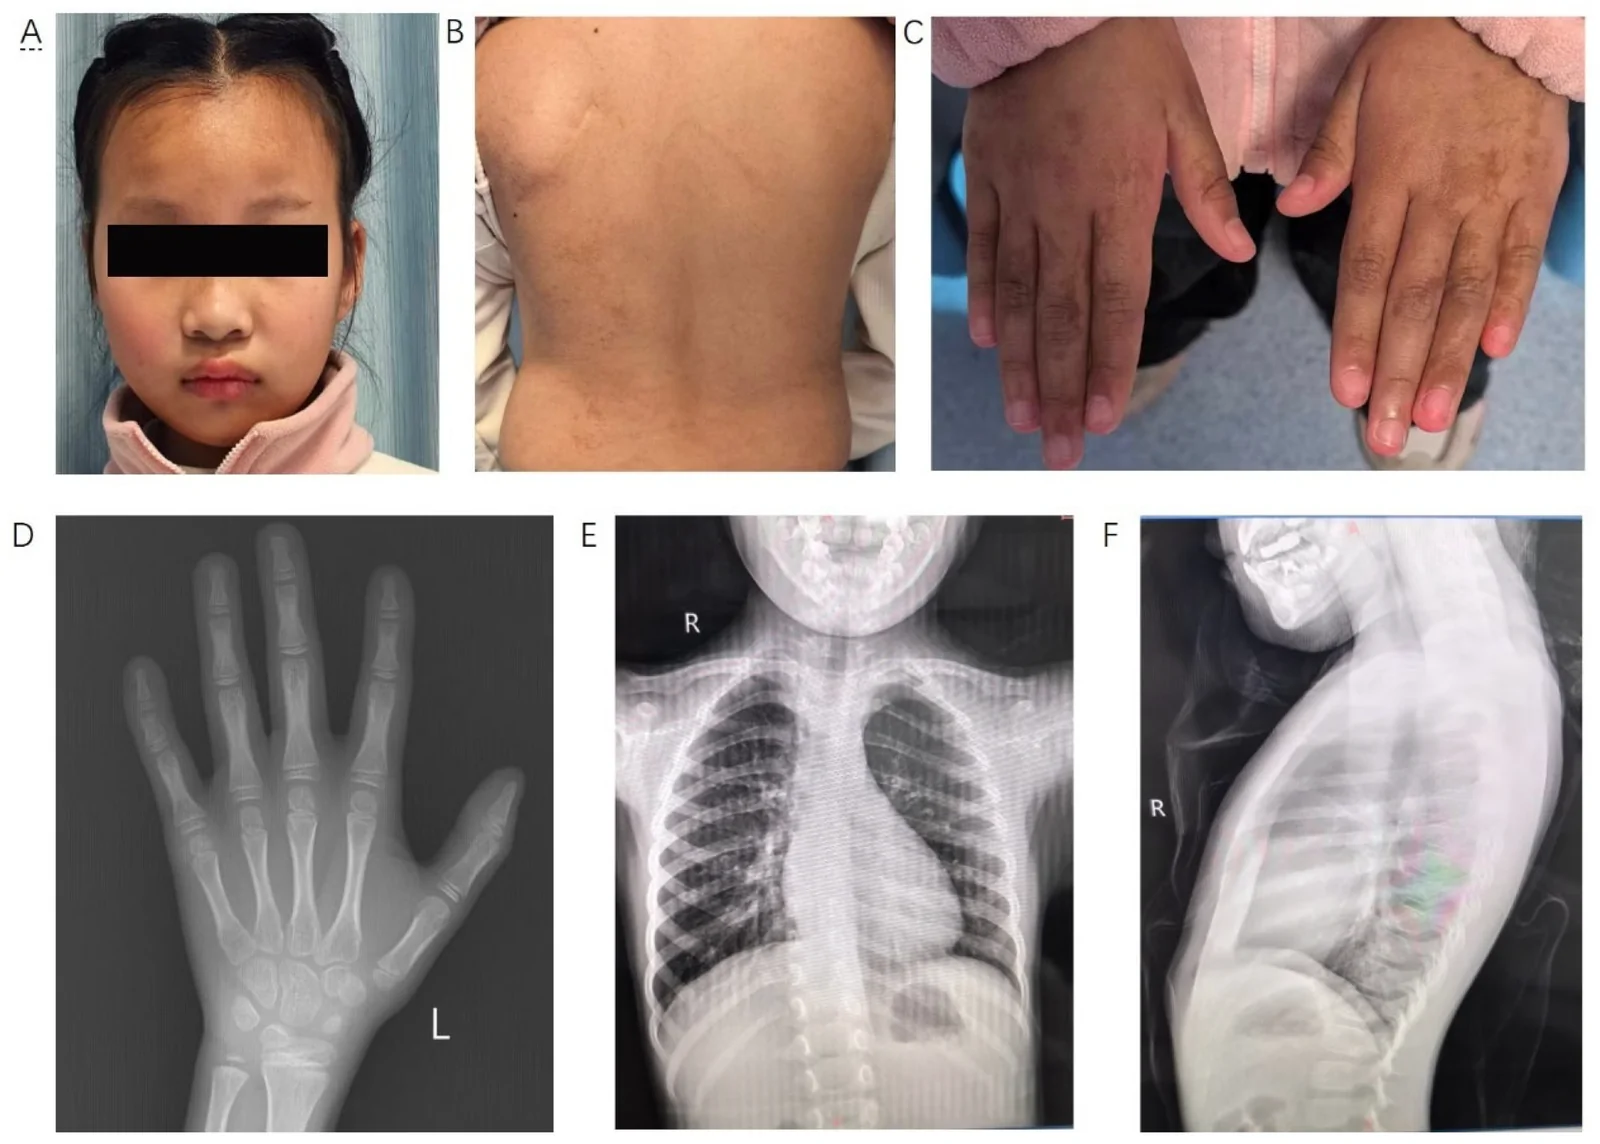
Clinical features of the 10-year-and-3-month-old female patient with mosaic trisomy 14 and severe short stature. (A) Facial features including hypertelorism, a low nasal bridge, short philtrum, upslanted palpebral fissures, and facial asymmetry; (B) scoliosis, back hyperpigmentation, and postoperative scar from PDA surgery; (C) small hands and pigmentary abnormalities on the hands; (D) left hand X-ray showing delayed bone age (6 years 8 months at chronological age 10 years 3 months); (E,F) whole-spine X-ray anteroposterior and lateral views showing right-convex thoracolumbar scoliosis (Cobb angle 13°) and thoracic kyphosis (30°).
